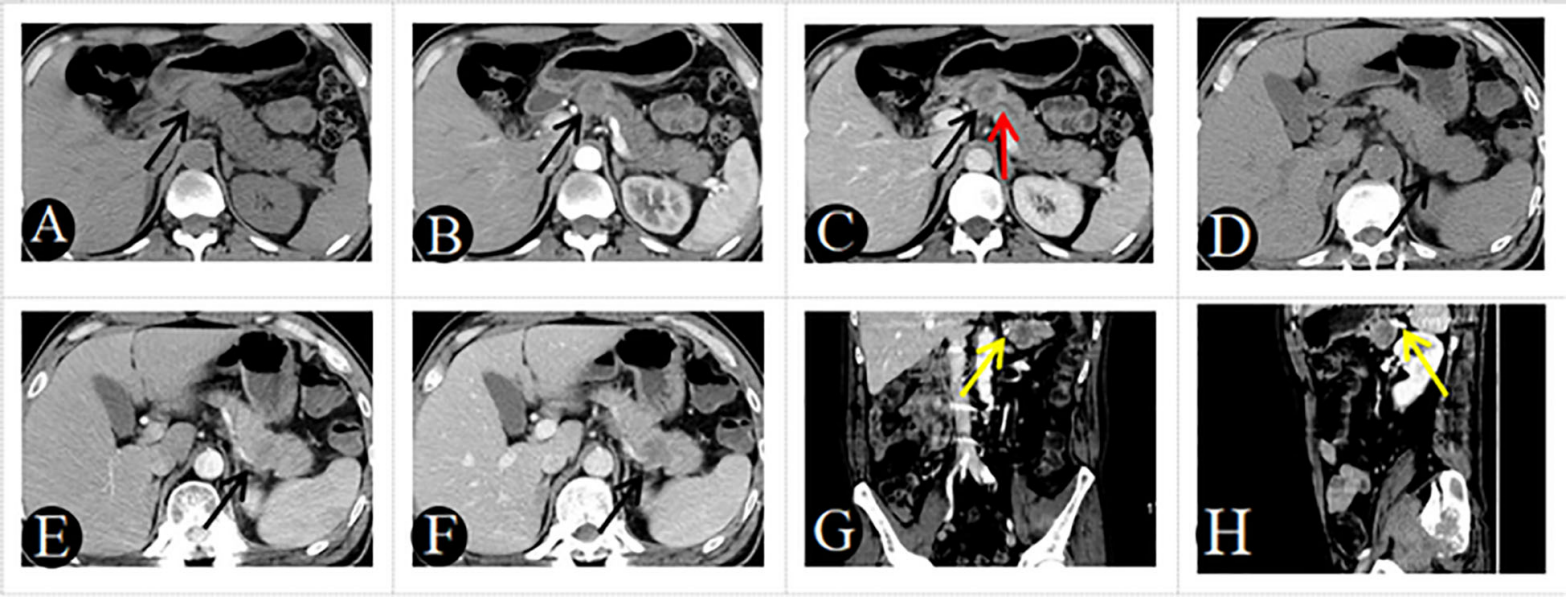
A 48-year-old man with PM-SCLC, absence of clinical symptoms, CA199 negative. Axial unenhanced (A), pancreatic parenchymal (B), and portal (C) phases CT images, demonstrated a low- or equal-attenuation nodules approximately 28mm in diameter in the neck of the pancreas (black arrow). The lesion showed gradual enhancement type, the typical rim enhancement and the pushed pancreatic duct (C, red arrow), with neither pancreatic parenchymal atrophy nor vascular invasion. A 51-year-old man with PM-SCLC, absence of clinical symptoms, CA199 negative. Axial unenhanced (D), pancreatic parenchymal (E), and portal (F) phases CT images, demonstrated a low- or equal-attenuation nodules approximately 30mm in diameter in the tail of the pancreas (black arrow). The lesion showed fast forward and backward enhancement type and the absence of vascular invasion (G, H, yellow arrow).
